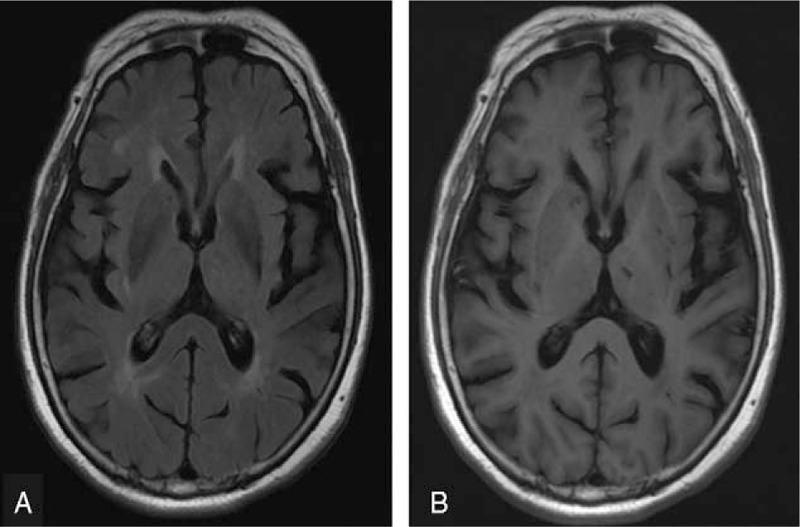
Brain magnetic resonance imaging of Case 1 showing small periventricular caps and subtle senile atrophy, which were both disproportionate to her cognitive deficits. (A) T2 fluid-attenuated inversion recovery image, axial view. (B) T1-weighted image, axial view.
